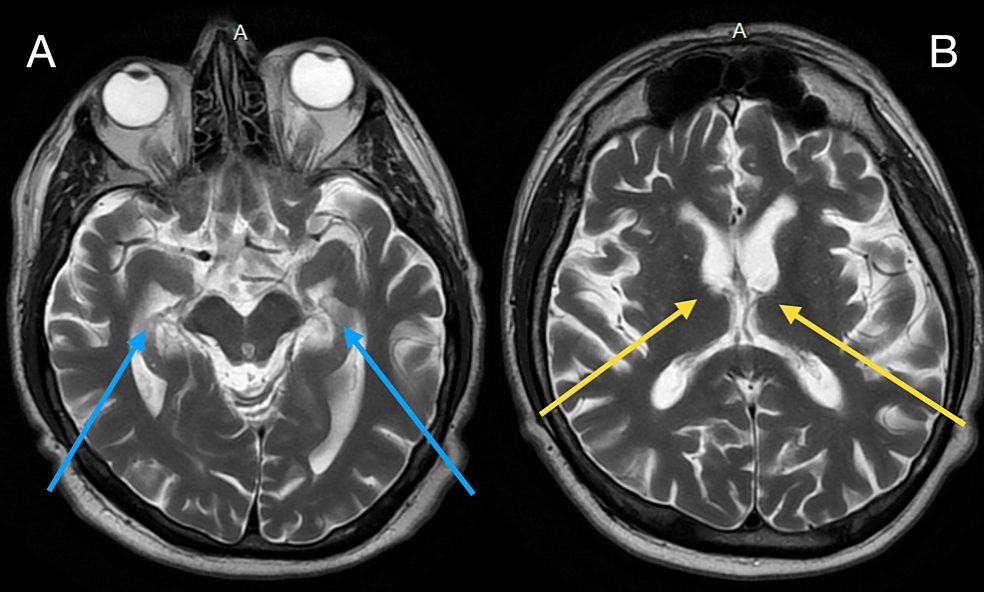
Brain MRI images of the patient MRI: Magnetic Resonance Imaging A) MRI displaying changes in hippocampal volume as depicted by the blue arrows. B) MRI showing changes in lateral ventricles volume as depicted by the yellow arrows.
